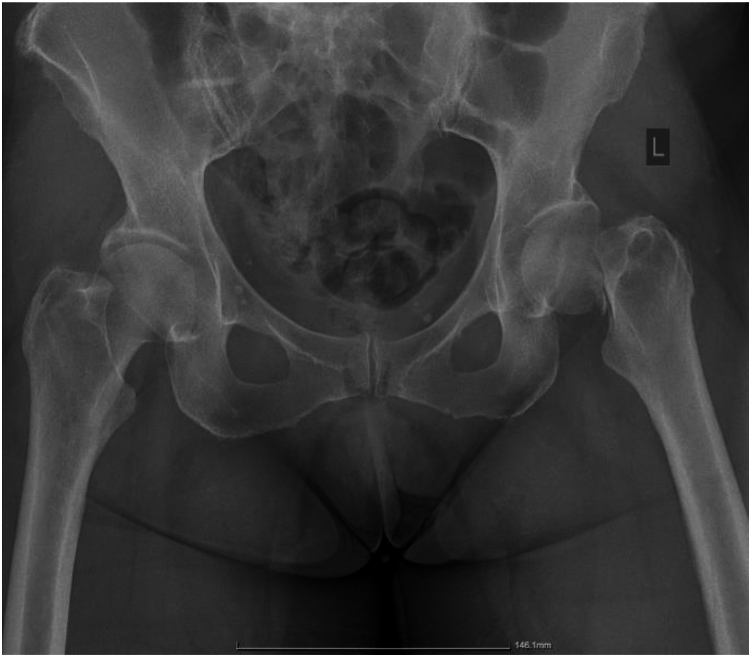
Anteroposterior X-ray of the pelvis, revealing a Garden III subcapital hip fracture.
